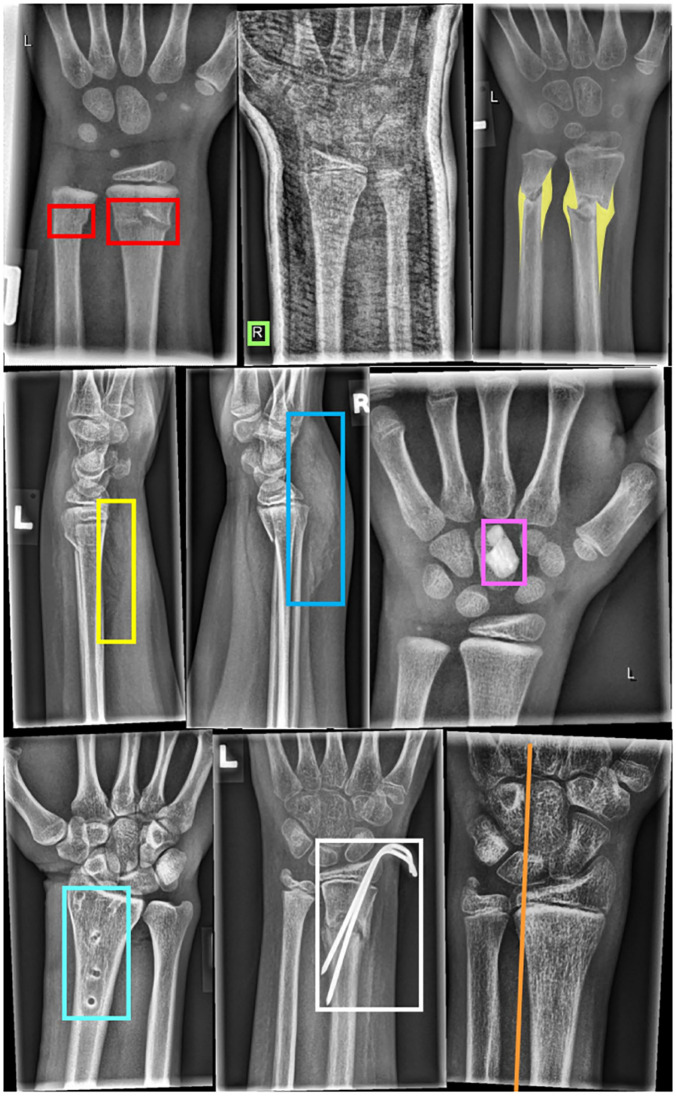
Examples of different objects labeled by human experts. From left to right in the first row are: Fracture, Text, Periosteal reaction. From left to right in the second row are: Pronator quadratus sign, Soft tissue swelling, Foreign body. From left to right in the last row are: Bone abnormality, Metal, and Axis. The X-ray in the middle of the first row shows a plaster cast. The images on the far right of the first and third rows are labeled as osteopenia.
